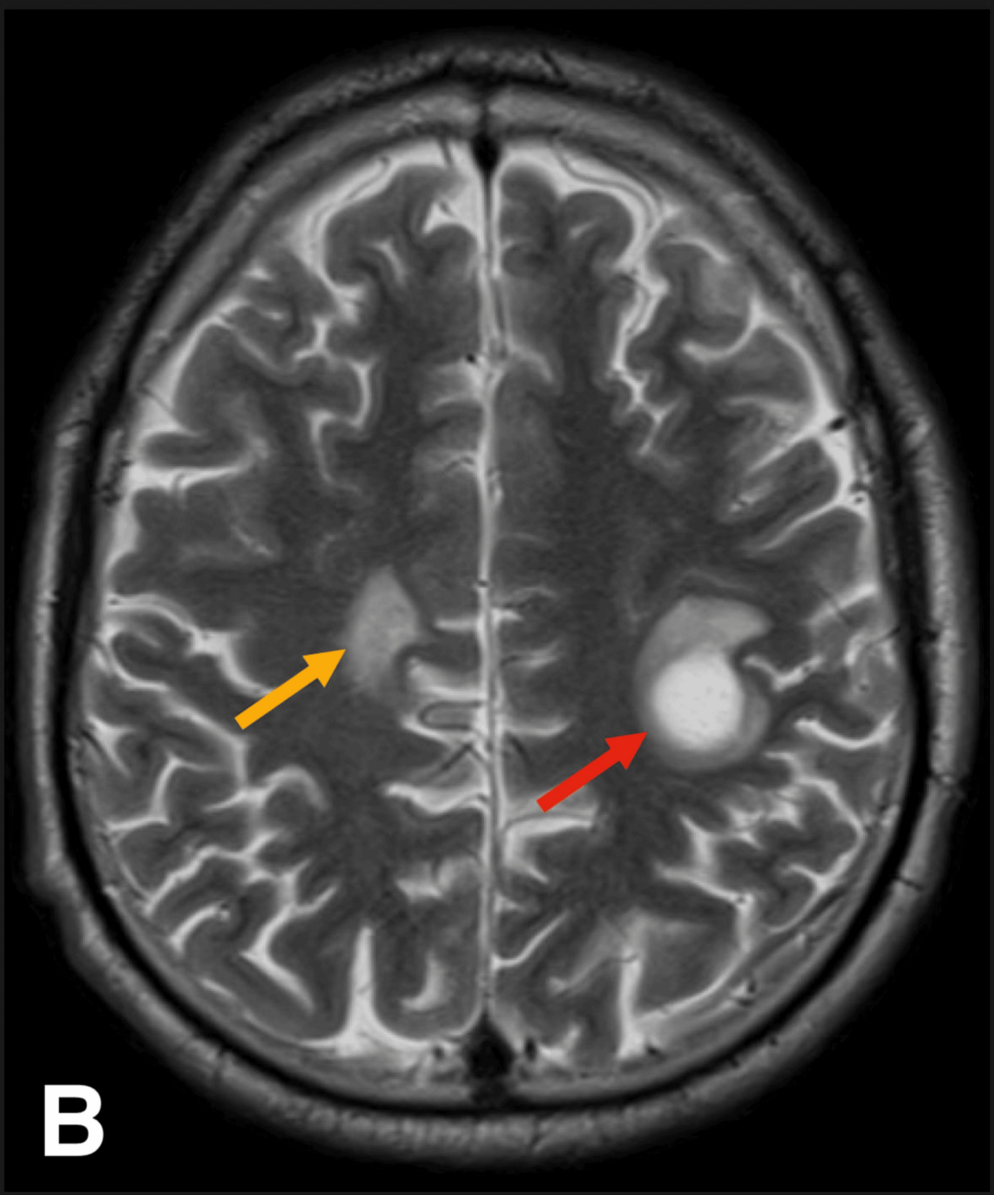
Brain MRI showing multiple focal lesions, including a 2-cm lesion in the left dorsal frontal lobe (red arrow) with partial ring enhancement
